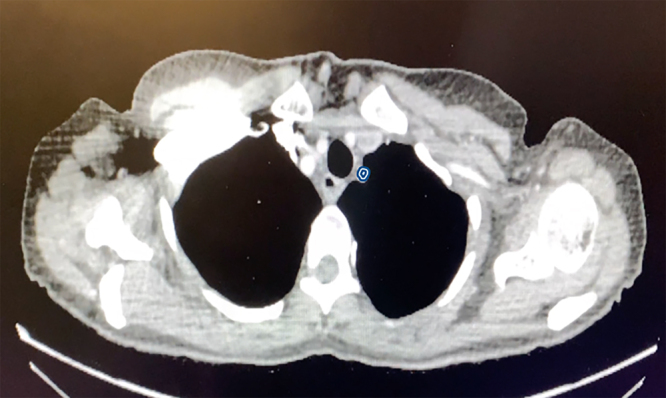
Axial contrast-enhanced computed tomography images of a 55-year-old woman (the subject of the case study) taken at approximately the level of T2. The image demonstrates a left-sided aberrant subclavian artery, highlighted in blue.
